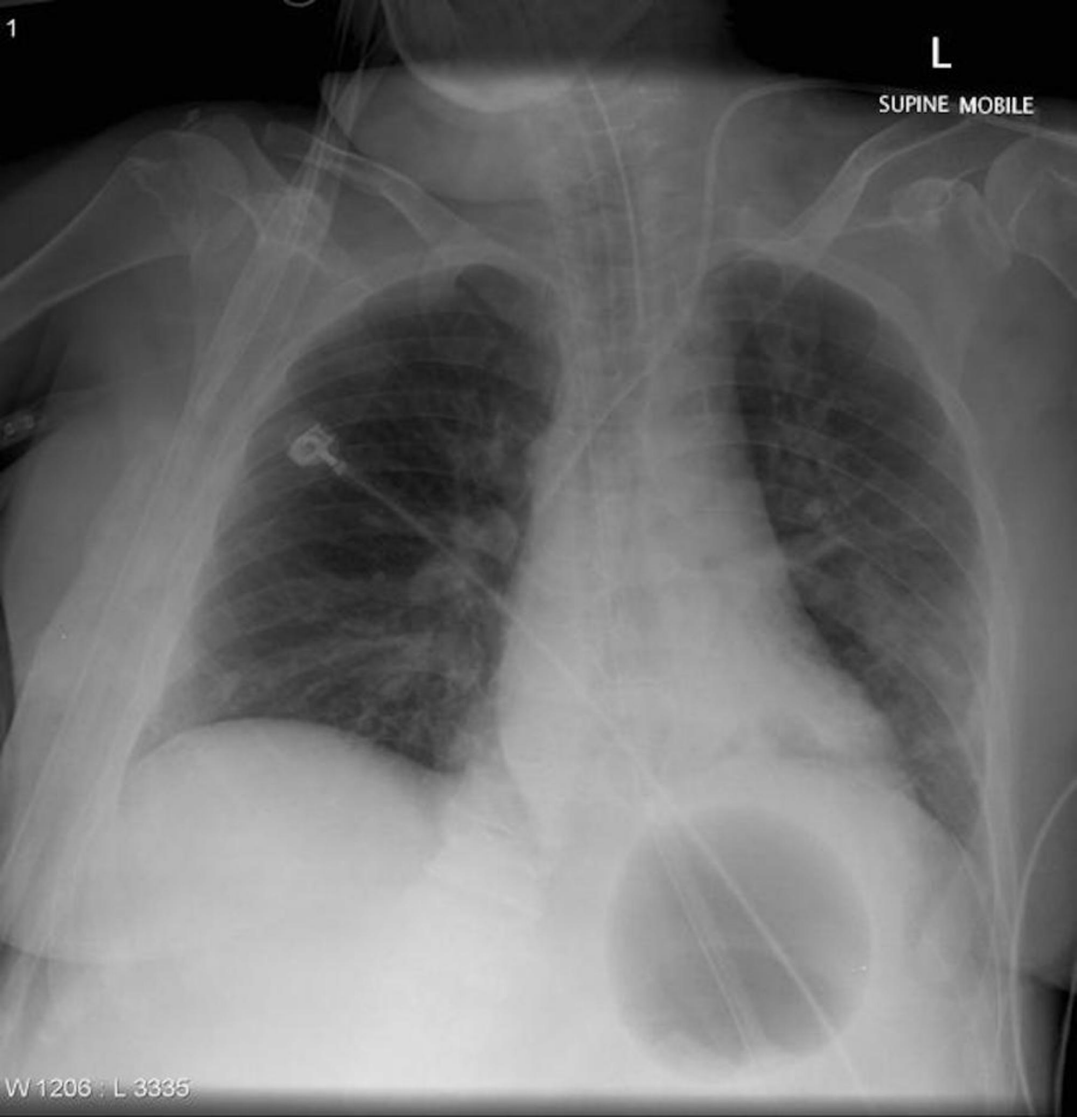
A supine chest X-ray demonstrating correct placement of a Sengstaken-Blakemore© tube (Case courtesy of A.Prof Frank Gaillard, Radiopaedia.org, rID: 12118).
